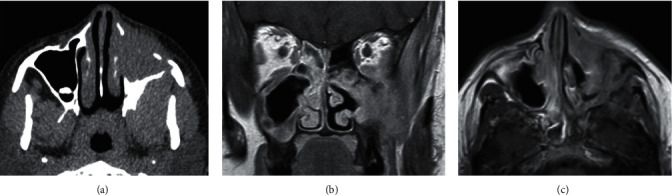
IgG4 disease. A 31-year-old female presented with epistaxis and medial-gaze diplopia. (a) Axial unenhanced CT shows soft tissue opacification of the left maxillary antrum with destruction of the antral walls and lateral pterygoid plate. (b) Coronal and (c) axial contrast-enhanced T1W demonstrates extension of the abnormally enhancing soft tissue (with restricted diffusion, not shown) into the orbit, pterygopalatine fossa, and involvement of the left infraorbital nerve.
